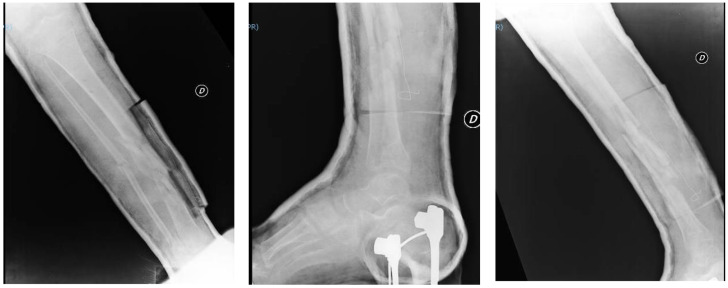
Radiograph of the right leg and ankle after the new treatment performed in October 2016, including the removal of the external fixator and packaging of the pin-femoral-podalic appliance incorporating a trans-calcaneal traction bracket.
